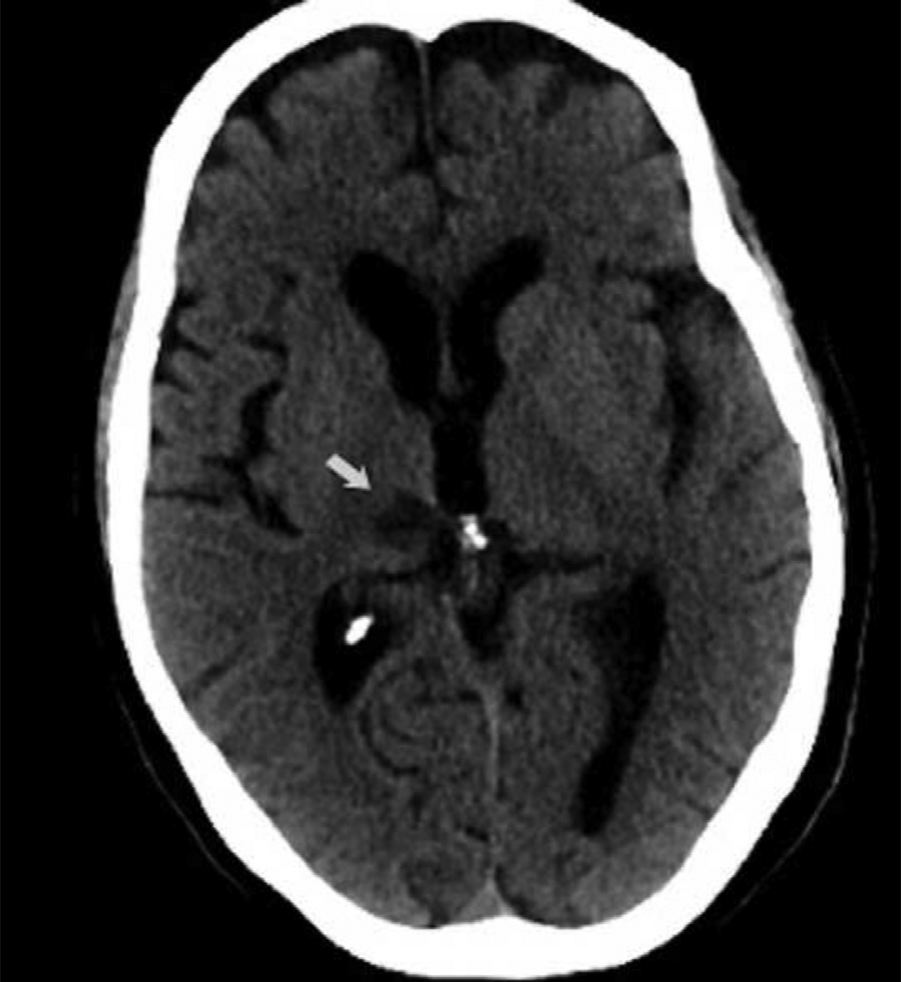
CT scan shows a hypodense area in the right thalamus.
